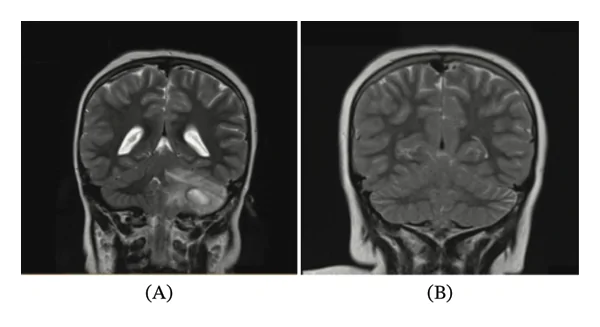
MRI head with (left) and without (right) IV contrast. (A) Approximately 2 cm, irregularly enhancing, cystic mass within the left cerebellar hemisphere with associated surrounding edema, regional mass effect, and approximately 6 mm midline shift. The findings are nonspecific, especially given the lack of clinical and serological markers for infection, but include infection, an inflammatory process, or neoplasm, including a variant appearance for pilocytic astrocytoma. (B) Resolution of the left cerebellar abscess 2.5 months after completion of the antibiotic course.
